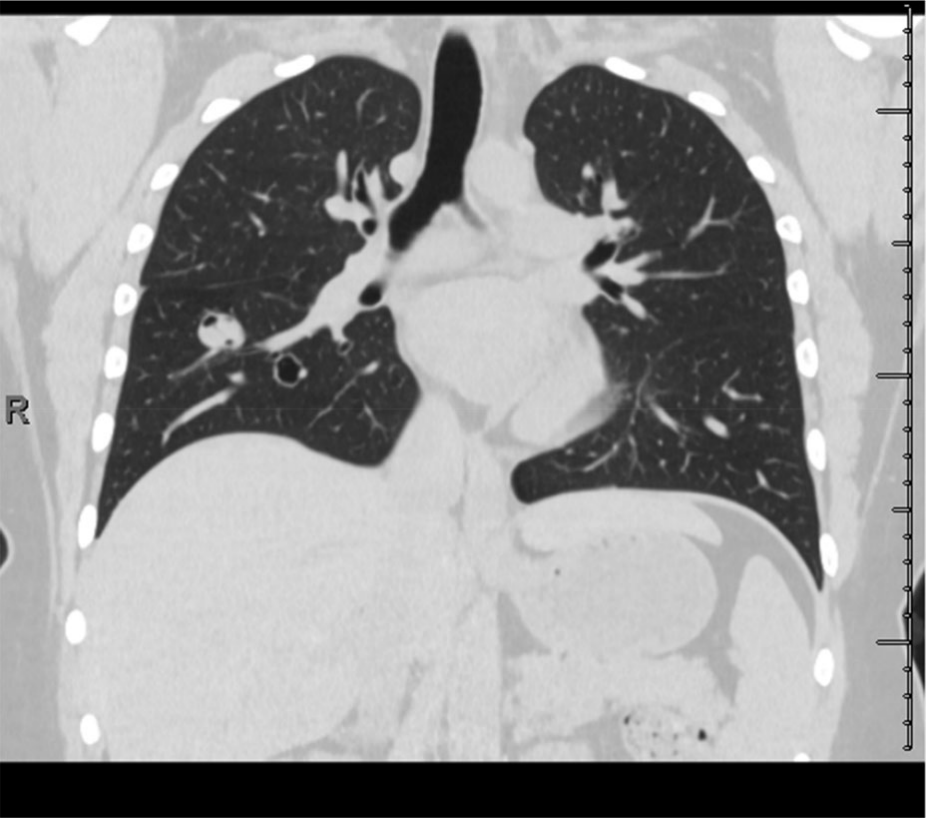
The figure shows a computed tomography (CT) scan of the patient's chest prior to starting treatment with bevacizumab. The CT chest shows a new 14 mm lobulated pulmonary nodule abutting the known right lower lobe cyst, a new multi‐cystic lesion in the right lower lobe.
